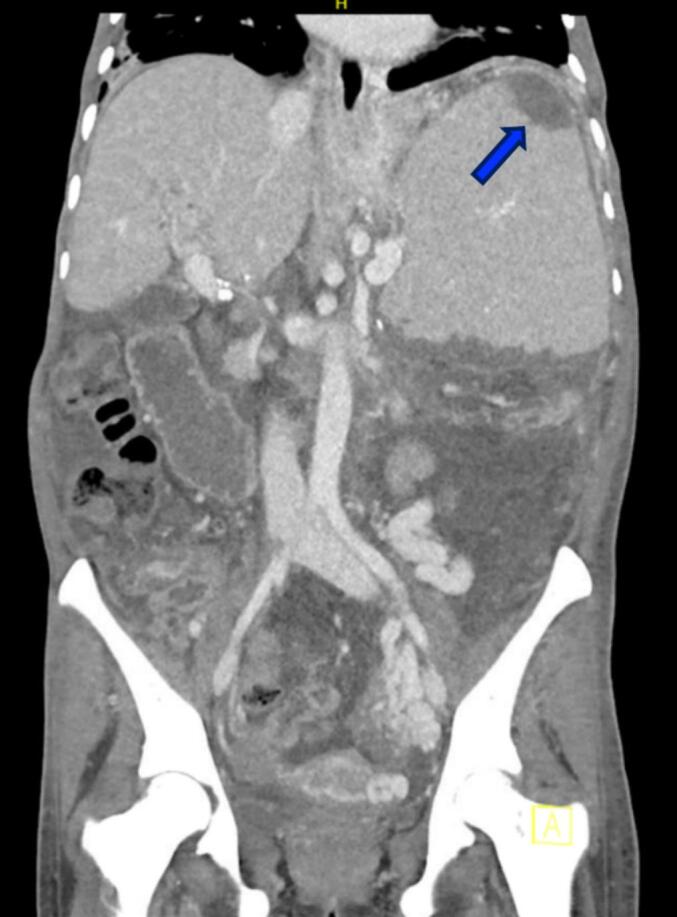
Post-operative CT of the abdomen and pelvis 5 days post open partial splenectomy assessing volume and supply to splenic remnant. A small focal infarct (blue arrow) is noted to the superior pole of the remnant spleen.
